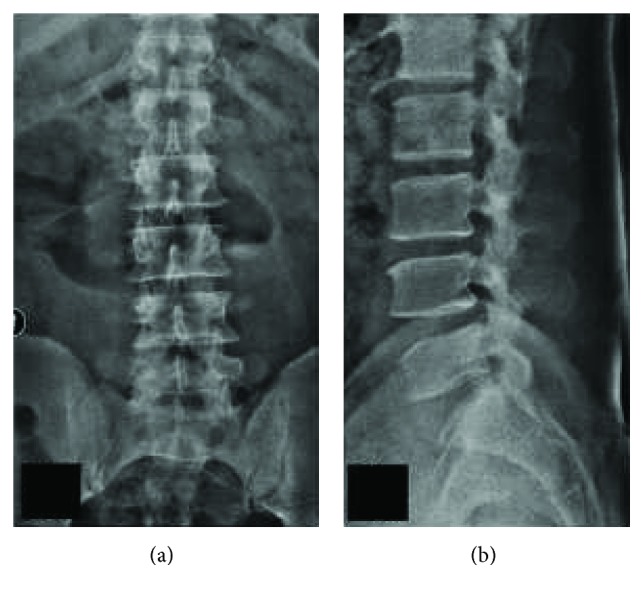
(a) and (b) Minimal spondylotic changes seen in plain radiography of the spine with no features of gross instability.
